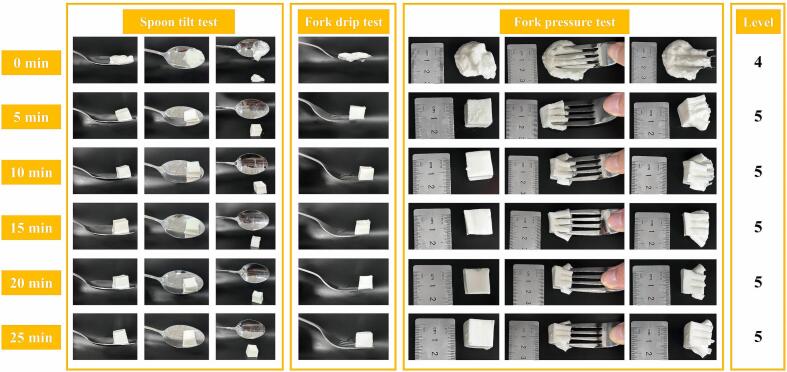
International Dysphagia Diet Standardisation Initiative (IDDSI) of Pickering emulsion gels stabilized by water-dispersible PSE-like myofibrillar protein microgel particles (MMPs) prepared with different ultrasound times (0, 5, 10, 15, 20, and 25 min).
